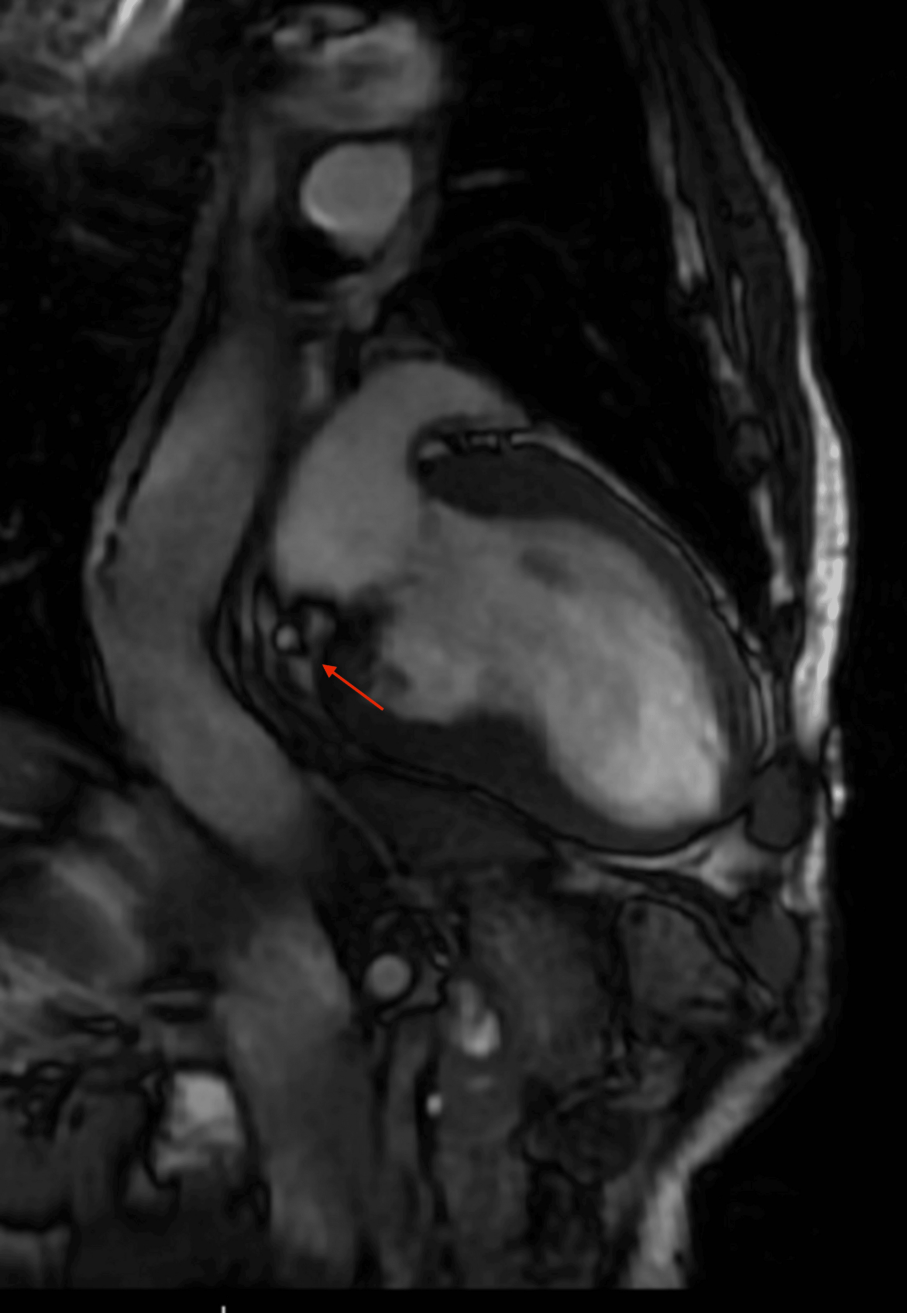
Cardiac MRI (coronal view) showing a mass (red arrow)
